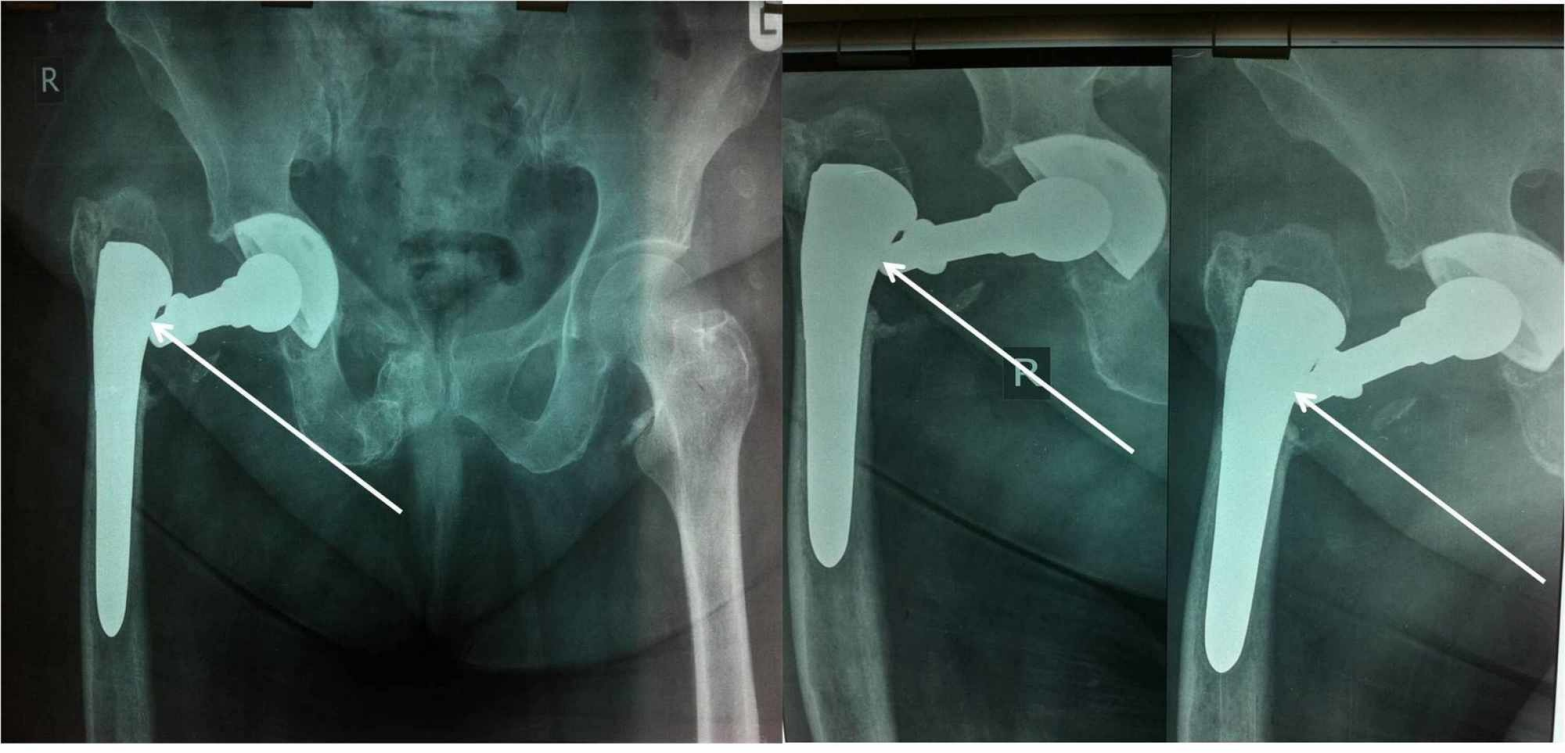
Radiographs show the dissociation of the femoral neck-stem interface after the fall (white arrows).
